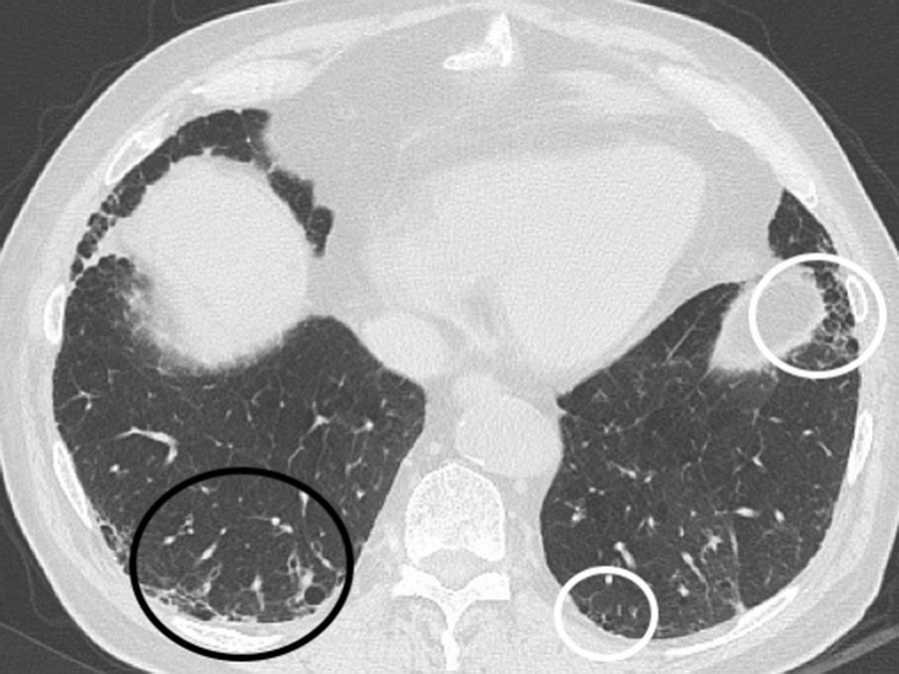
Axial CT image without contrast (slice thickness of 1 mm) of a 68-year-old gentleman shows traction bronchiectases (*white circle*) and some cystic airspaces with thick fibrous walls (*black circles*), which represent fibrotic parenchymal changes
